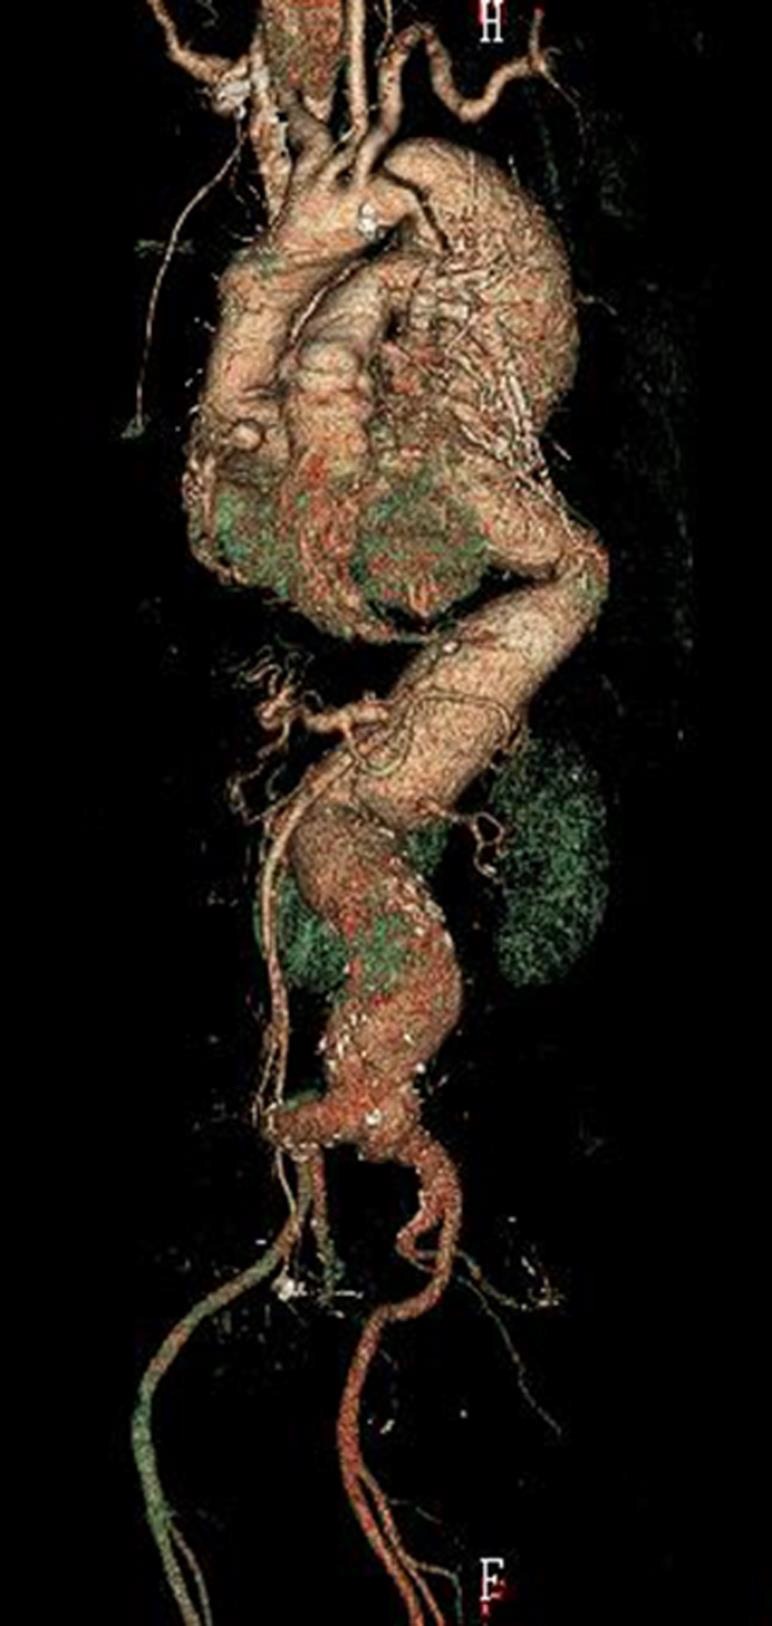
Follow‐up CT 18 years after the initial surgery. The descending and abdominal aortae with a pseudolumen are enlarged.
